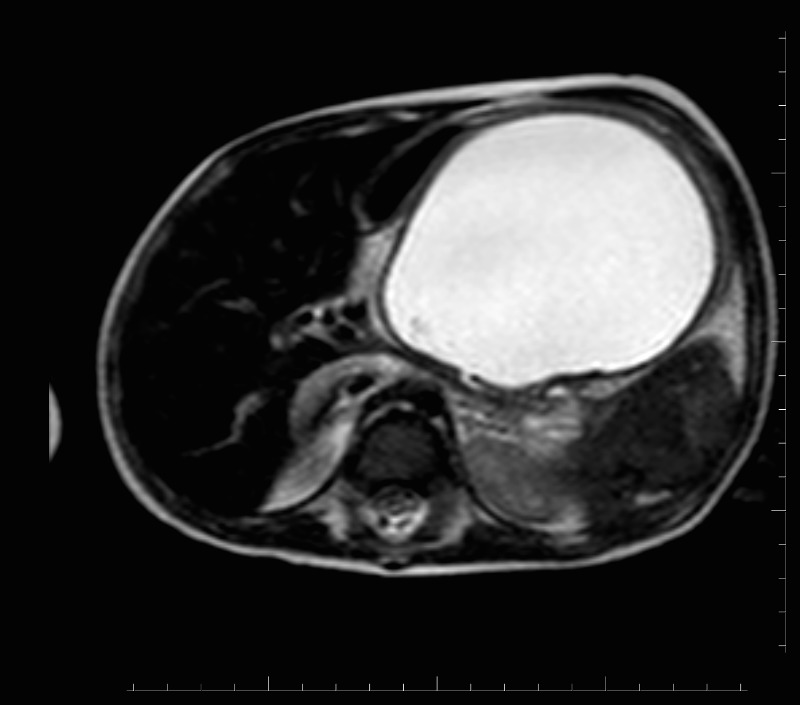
Figure 1: MRI showing a large cystic collection of fluid in front of body and tail of pancreas, pushing the stomach anteriorly.
